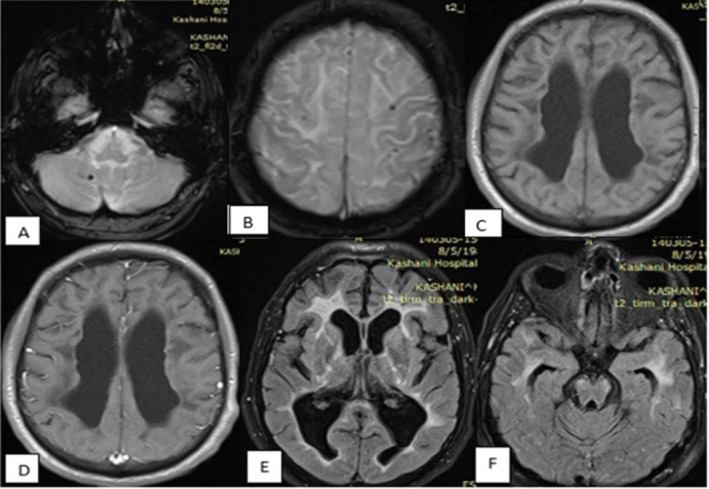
Brain MRI of the patient at admission. (A, B) Gradient echo sequences showing focal low signal intensities suggestive of cerebral microhemorrhages. (C) T1‐weighted image demonstrating hypointensities in the periventricular and subcortical regions. (D) Post‐contrast T1‐weighted image showing no pathological enhancement. (E, F) FLAIR sequences revealing confluent hyperintensities in the periventricular and subcortical white matter, anterior temporal lobes, and external capsule, consistent with diffuse leukoencephalopathy and associated brain atrophy.
